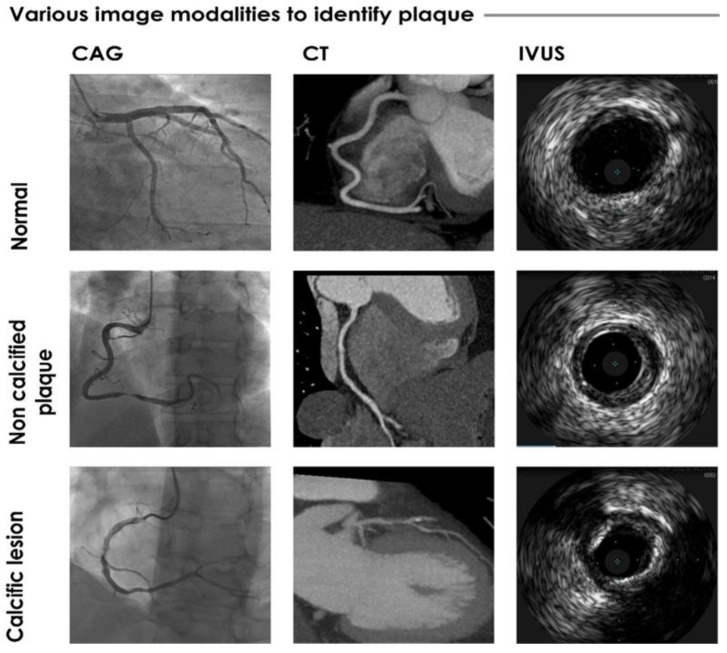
Sample images from invasive CAG, CT, and IVUS imaging techniques.
